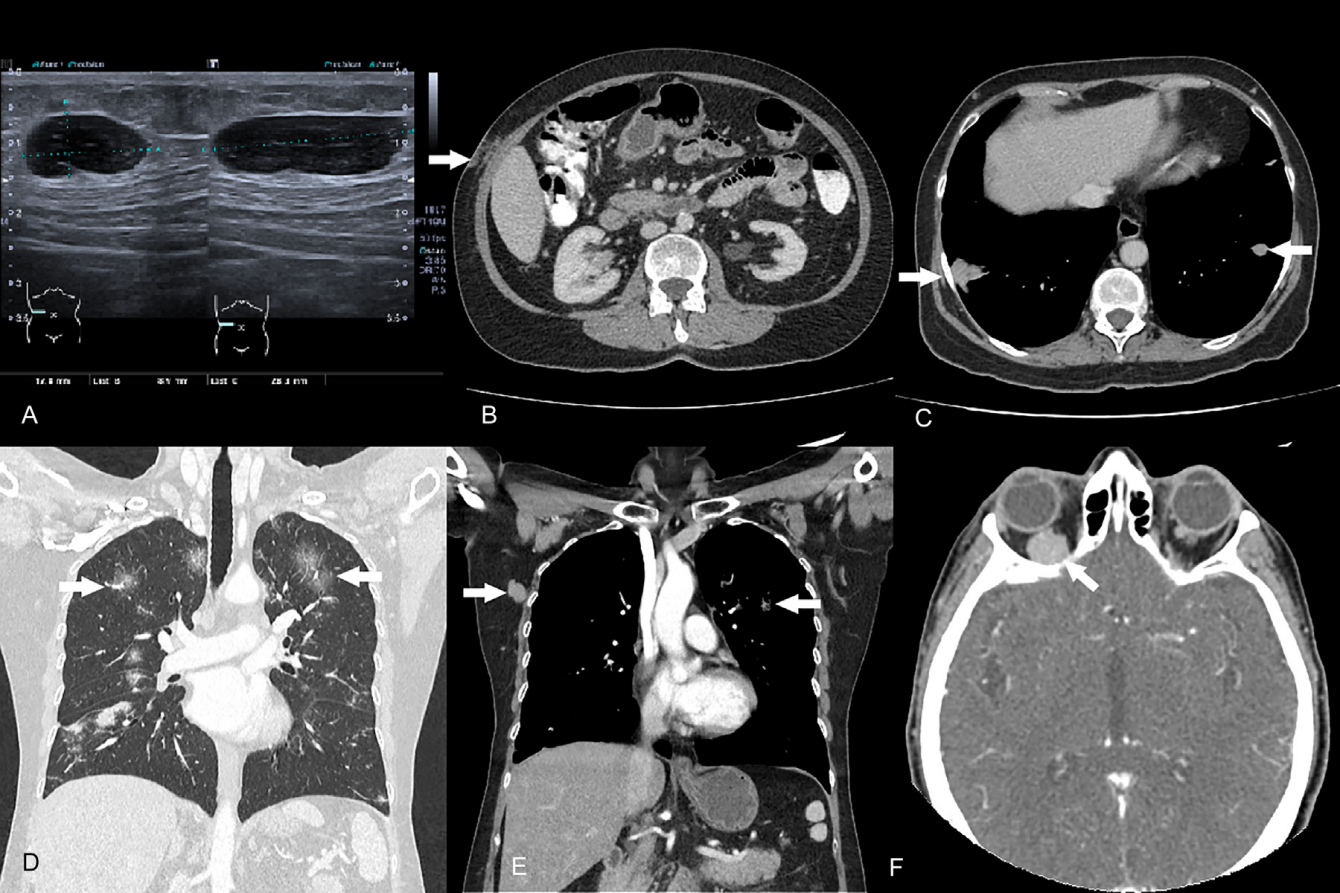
Demonstrative findings highlighted with white arrows. (A) Ultrasound of the soft tissues in the right hypochondrium displaying hypoechoic subcutaneous nodules with distinct borders and minimal flow on color Doppler. (B) Contrast-enhanced tomography of the chest with a coronal window reconstruction for the lungs, showcasing multiple central and peripheral subsolid nodules with a ground glass halo effect. (C) Mediastinal window reveals right axillary adenomegaly with no other lymph node involvement detected. (D) Contrast tomography of the abdomen shows subcutaneous nodules in the right hypochondrium region. (E) The lower base of the thorax displays solid pulmonary and subcutaneous nodules. (F) Neck tomography highlights an orbital soft tissue mass with right intraconal enhancement encompassing the optic nerve.
